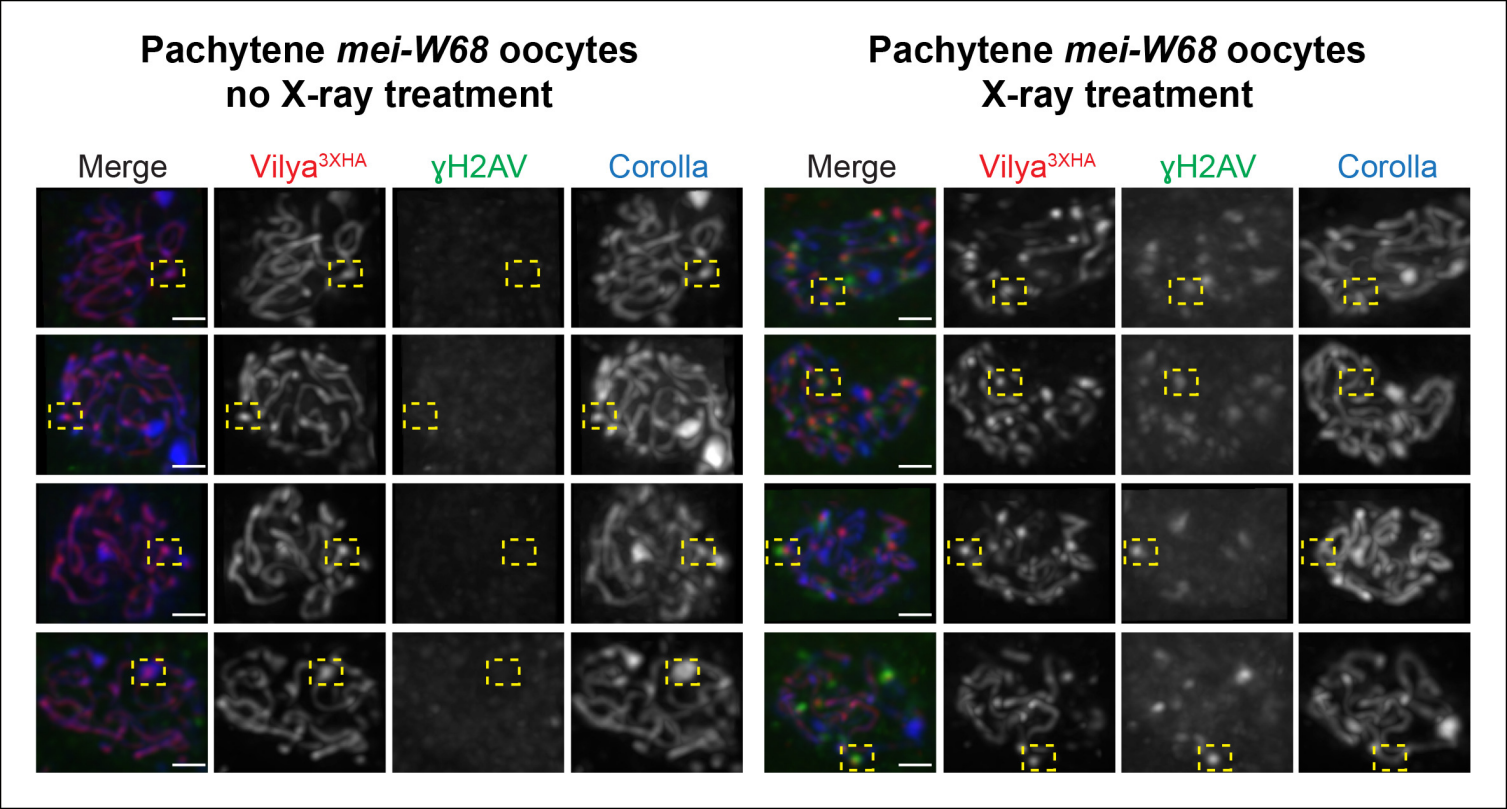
Some DSBs created by X-ray can recruit Vilya3XHA to discrete
